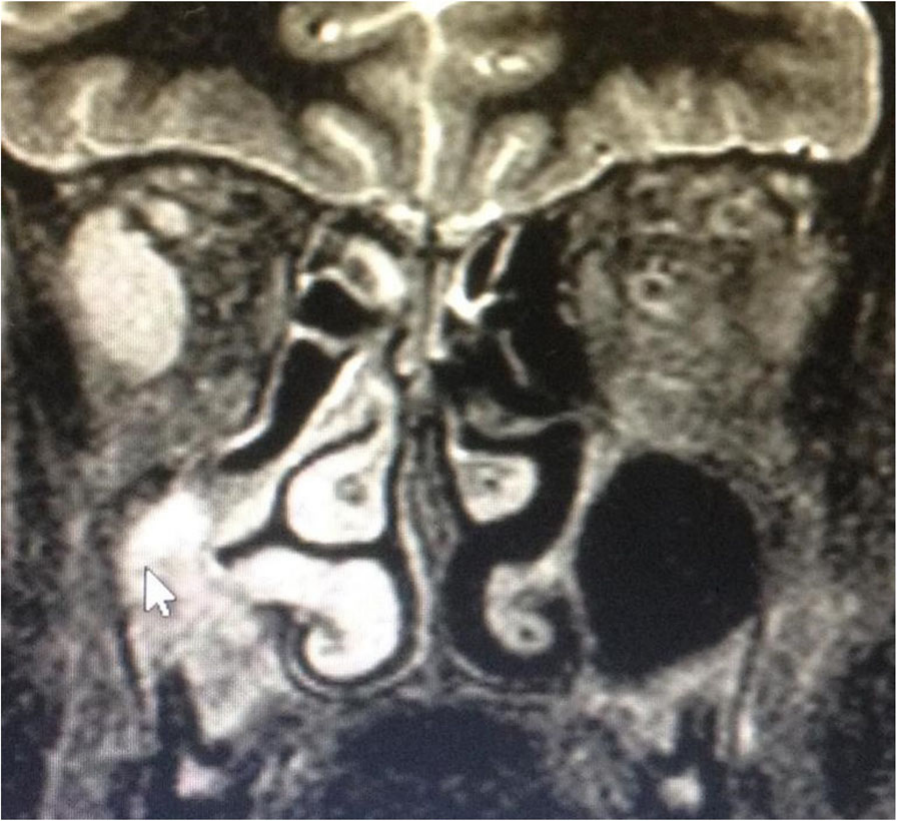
Preoperative MR imaging (coronal view): right intraorbital intraconic abscess and signs of acute maxillary sinusitis
